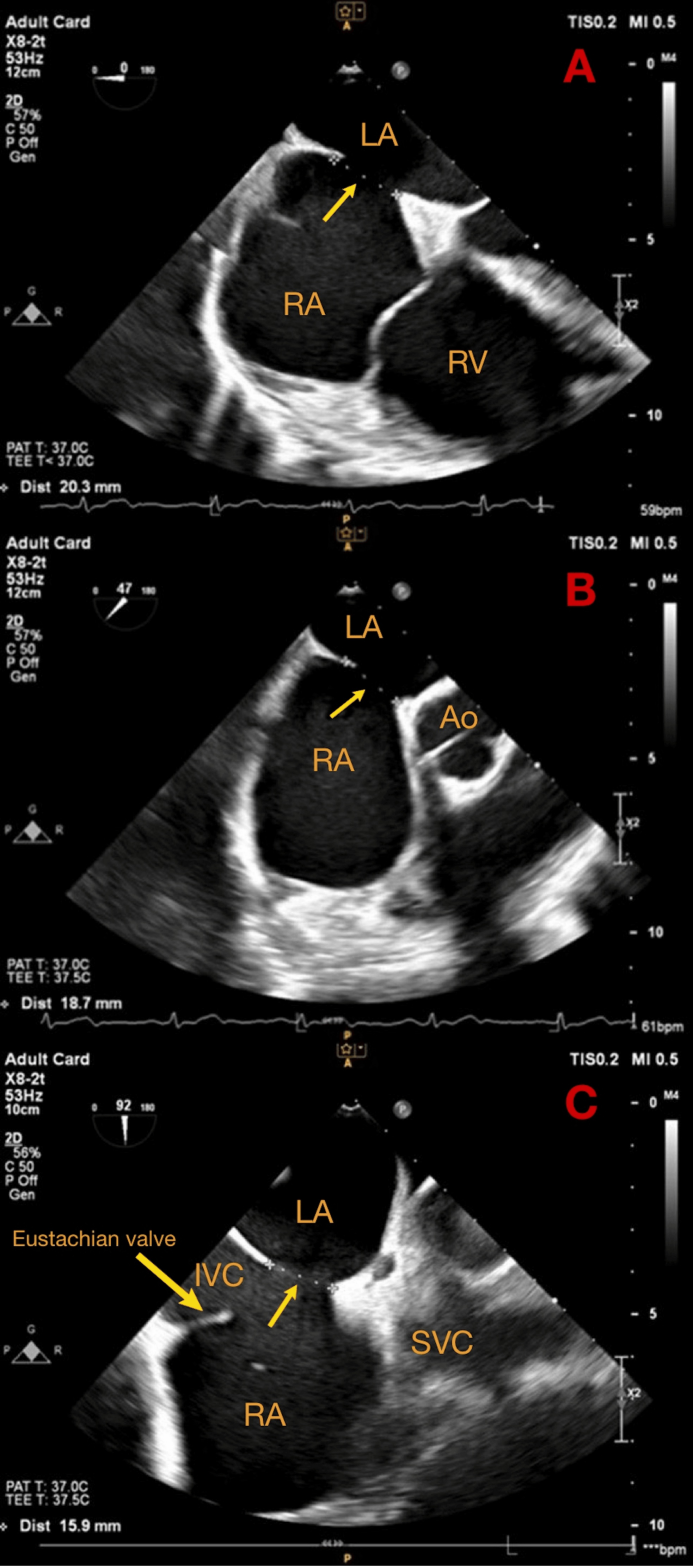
Transesophageal echocardiography (TEE) with four-chamber view (A), short axis view (B), and bicaval view (C) at 0°, 47°, and 90°. RV: right ventricle; RA: right atrium; LA: left atrium; Ao: aorta; SVC: superior vena cava; IVC: inferior vena cava
